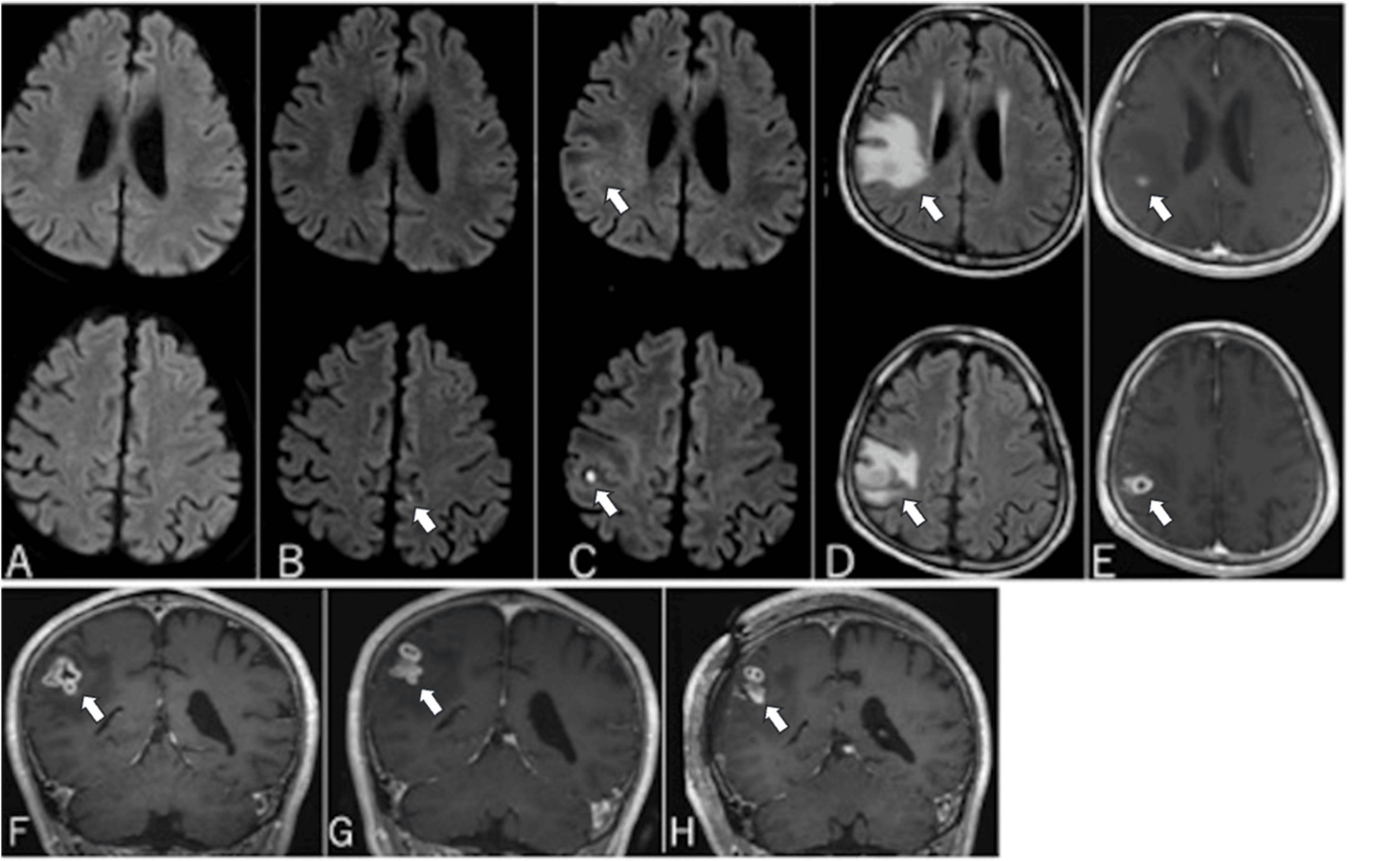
Cranial MRI (A-C: DWI, D: FLAIR, E-I: contrast-enhanced MRI). (A) Initial presentation with a transient ischemic attack (TIA); no abnormal findings were observed. (B) At 6 months after the initial visit, revealing cerebral infarction (arrowhead) in the left parietal lobe. (C) At 11 months after the initial visit, showing a hyperintense signal on DWI in the right parietal lobe (arrowhead), with findings atypical for cerebral infarction. (D) A FLAIR image was obtained showing extensive brain edema surrounding the lesion (arrowhead). (E) A contrast-enhanced MRI was obtained after admission, revealing a ring-enhancing lesion in the right parietal lobe (arrowhead). (F) Upon admission, with a contrast-enhancing lesion (arrowhead). (G) Immediately before biopsy, the contrast-enhancing lesion was slightly enlarged (arrowhead).(H) Post-biopsy MRI showed that a small amount of the lesion was removed by biopsy (arrowhead) and that there were no surgical complications. MRI: Magnetic resonance Iimaging, DWI: Diffusion-weighted imaging, FLAIR: Fluid-attenuated inversion recovery, TIA: Transient ischemic attack
